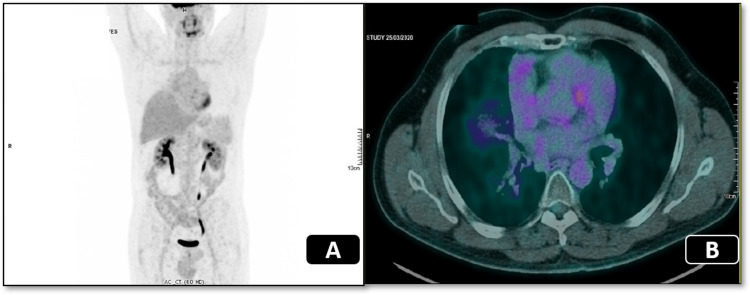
PET CT in March 2020 shows the tumor lesion disappearance (A - coronal plane; B - transversal plane).
